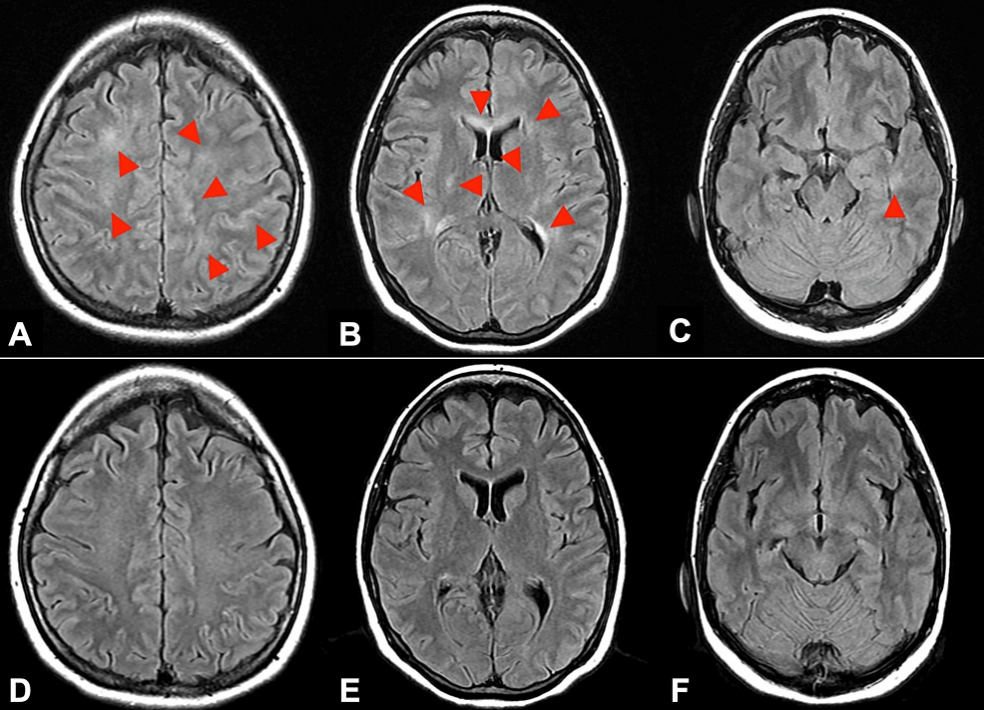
Brain MRI images of fluid-attenuated inversion recovery (FLAIR) before and after steroid pulse therapy. (A–C) were obtained before steroid pulse therapy. Brain MRI of the parietal level (A), basal ganglia level (B), and midbrain level (C) images showing hyperintensity of the subcortical white matter, deep white matter, and corpus callosum (red arrows). (D–F) were obtained after steroid pulse therapy. Each levels of brain MRI revealed almost complete reversal of previously observed high signals.
